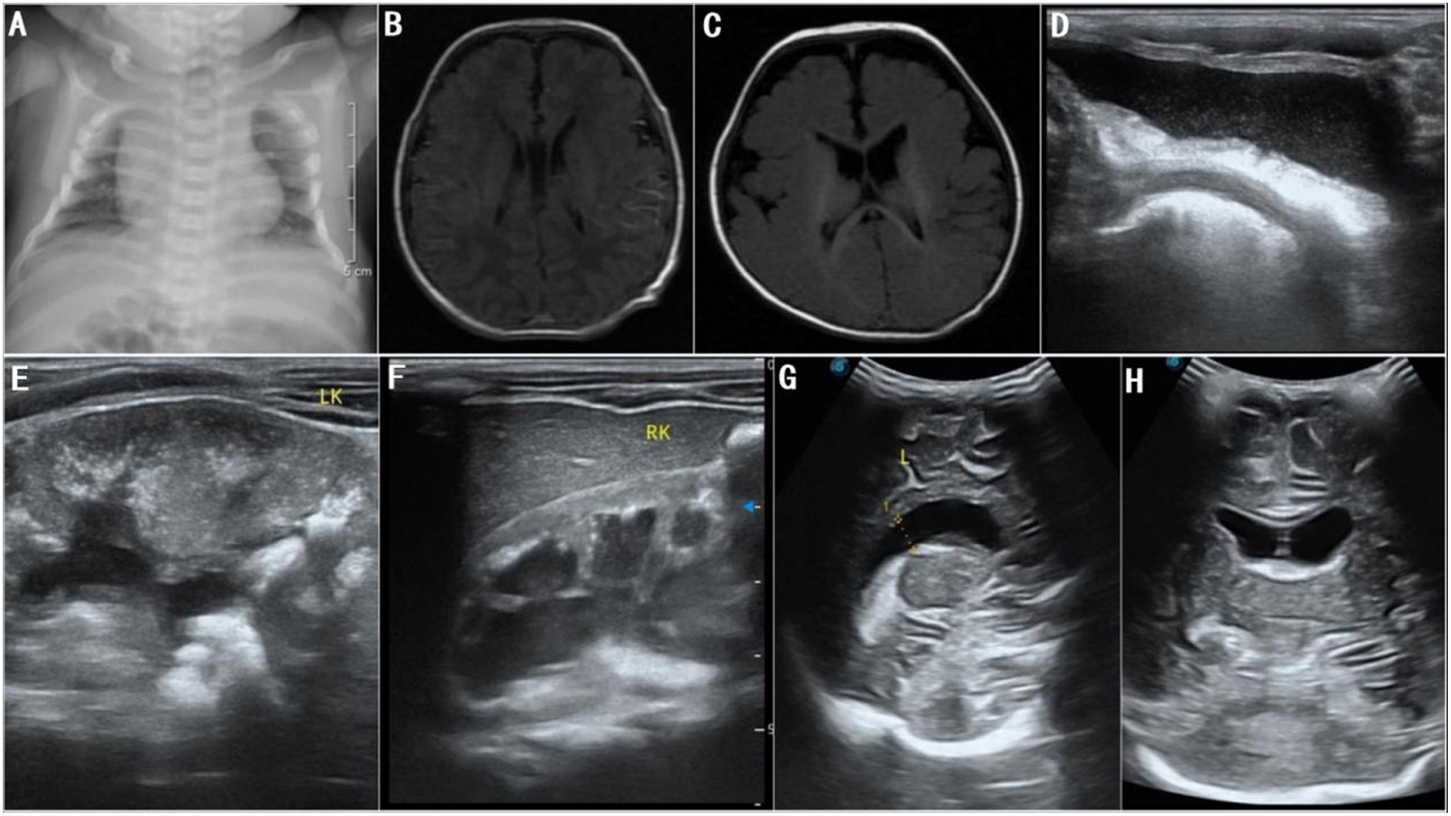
(A) Chest radiographs at 3 days after birth show broad bones from the 2^nd^ to the 5^th^ pair of anterior ribs and thoracic deformity; (B) brain MRI at 5 days after birth shows normal lateral ventricles; (C) brain MRI at 6 months after birth shows normal lateral ventricles; (D–F) color Doppler ultrasonography at 18 months after birth shows bilateral hydronephrosis, and deposition of calcium in bilateral renal parenchyma, bilateral renal pelvises, and bladder; (G,H) color Doppler ultrasonography at 18 months after birth shows widened bodies and anterior horns of lateral ventricles.
